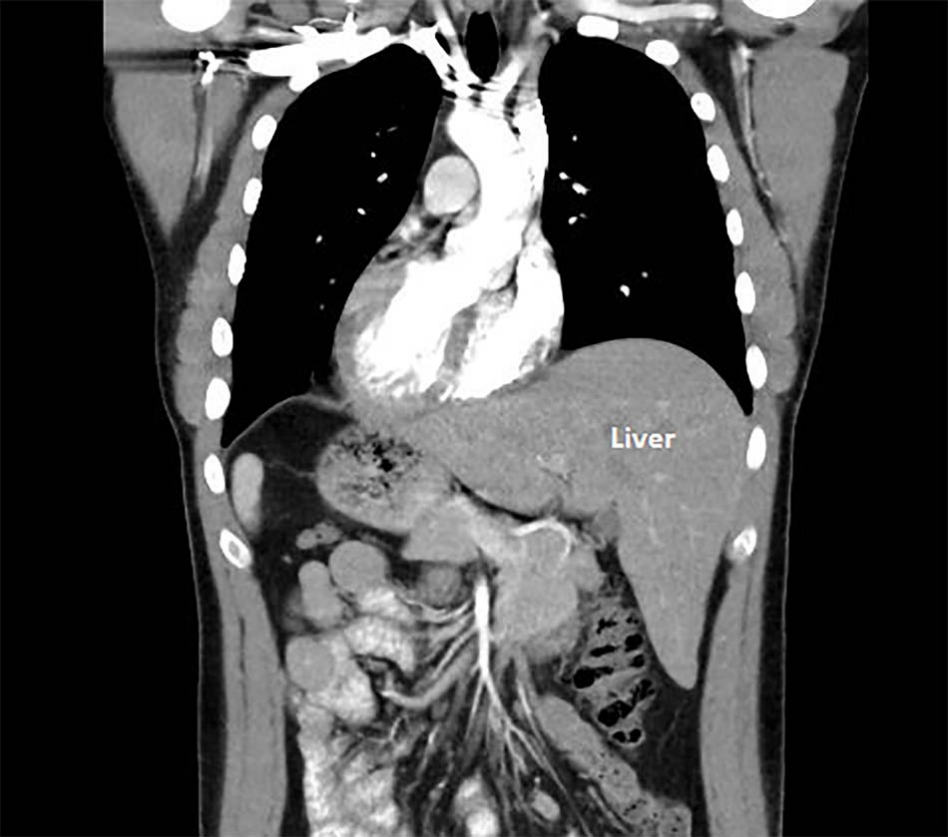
Coronal cross-section image of computed tomography scan.
